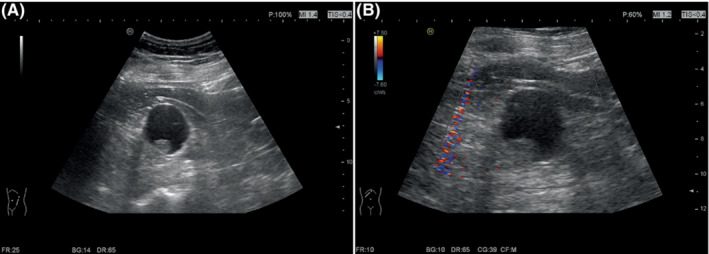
Ultrasonography of succinate dehydrogenase‐deficient renal cell carcinoma. (A) Grayscale ultrasound showed that the cystic‐solid mass was oval, well‐defined, and approximately 41 mm × 40 mm in size. (B) Color Doppler flow imaging showed that the solid component of the mass was isoechoic and approximately 16 mm × 9 mm in size without any blood flow signals.
